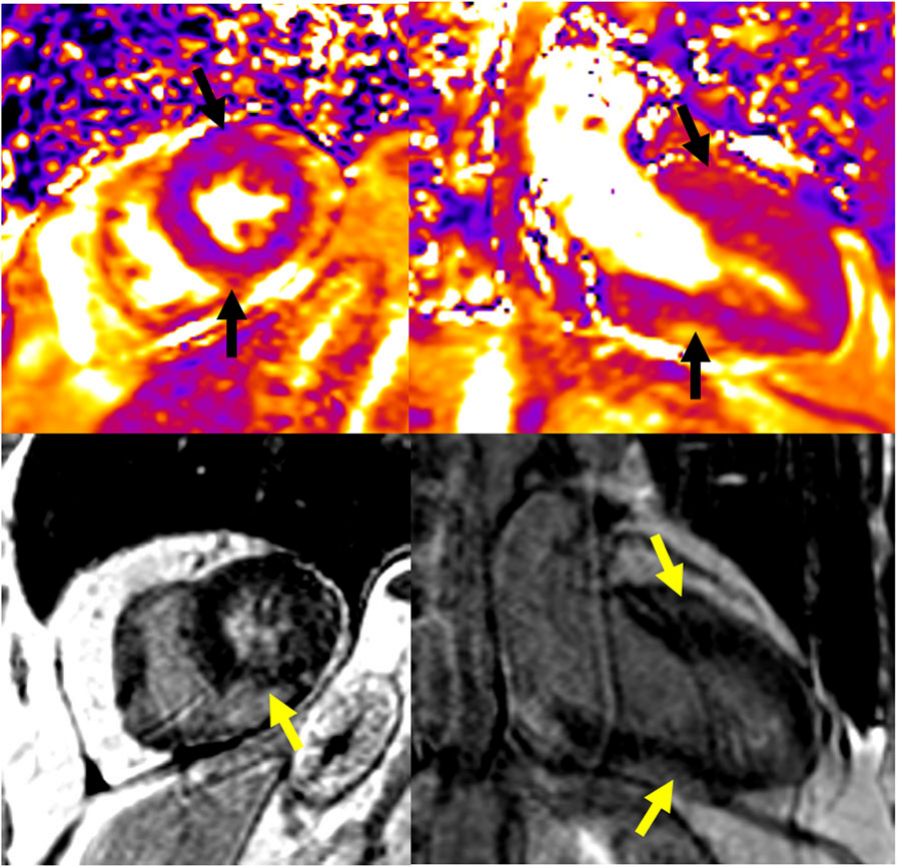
Cardiac MRI from Case 1: T2 maps (top row) demonstrate patchy focal areas of myocardial edema in the anterior wall and inferior wall (black arrows). Late gadolinium enhancement (LGE) images (bottom row) demonstrate patchy focal areas of scar (yellow arrows). These findings are consistent with myocarditis
